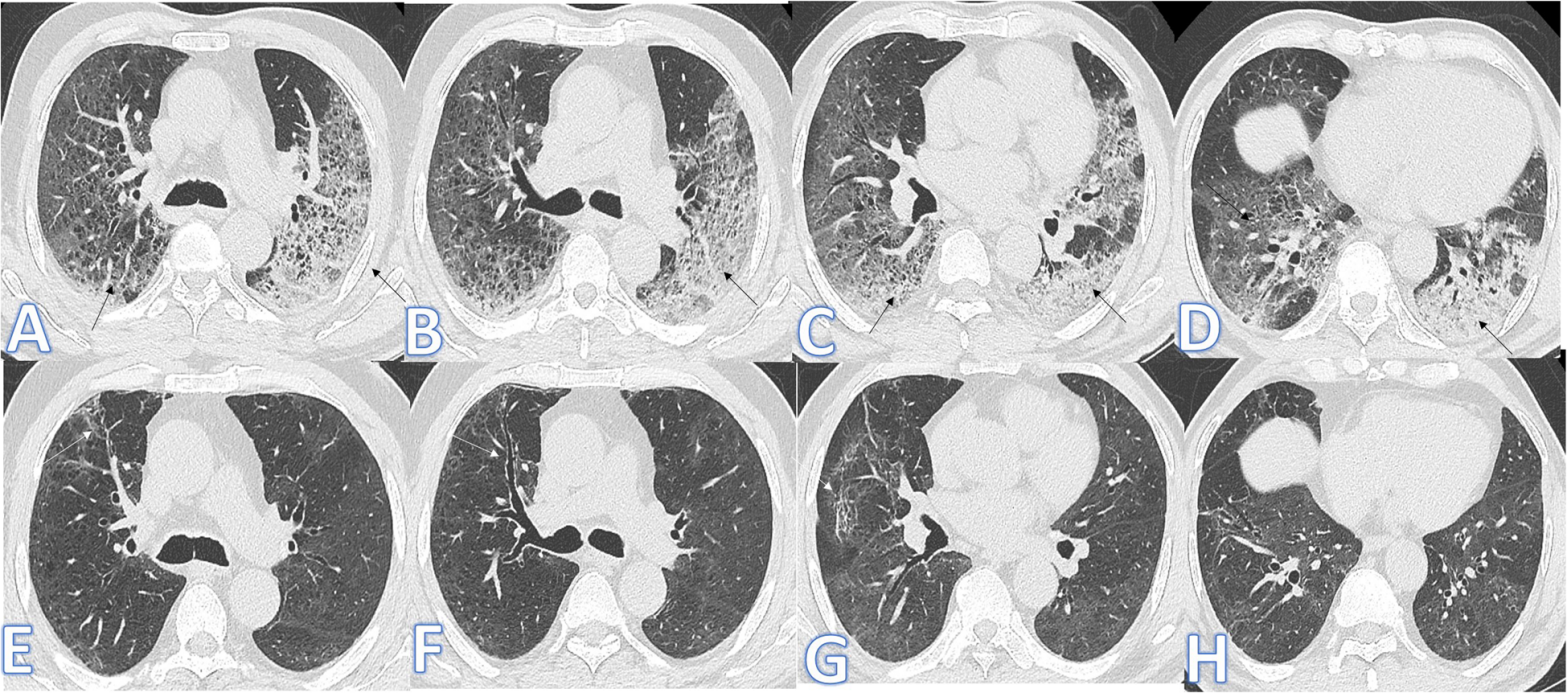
Sixty-four-year-old male: he has DM and HTN; peak CT severity score 17; consolidation/crazy-paving score 11. No ICU admission; laboratory results showed lymphopenia, high CRP, d-dimer, and serum ferritin. Steroid was given; length of stay during hospitalization is 20 days. Peak CT (a–d) showed GGO with interstitial thickening giving a crazy-paving appearance (arrows). Follow-up CT 45days after discharge (e–h) showed almost resolution of the previous with residual fibrosis with parenchymal bands, coarse reticular pattern, and mild traction bronchiectasis at the anterior segment of the right upper lobe and lateral segment of the right middle lobe (arrows)
